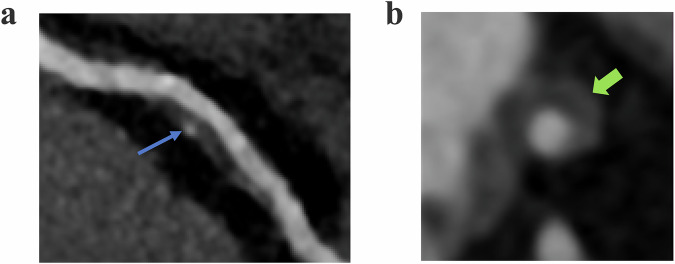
Representative examples of high-risk coronary plaques on coronary CT angiography. a Coronary CTA (70-year-old woman) shows a mixed plaque in the proximal left anterior descending (LAD) artery that is predominantly non-calcified; it meets three high-risk criteria—spotty calcification (thin blue arrow), low attenuation (< 30 HU), and positive remodeling. b Coronary CTA (67-year-old man) demonstrates a non-calcified plaque in the proximal LAD exhibiting the napkin-ring sign (thick green arrow), characterized by a central low-density core surrounded by a higher-attenuation rim adjacent to the lumen
